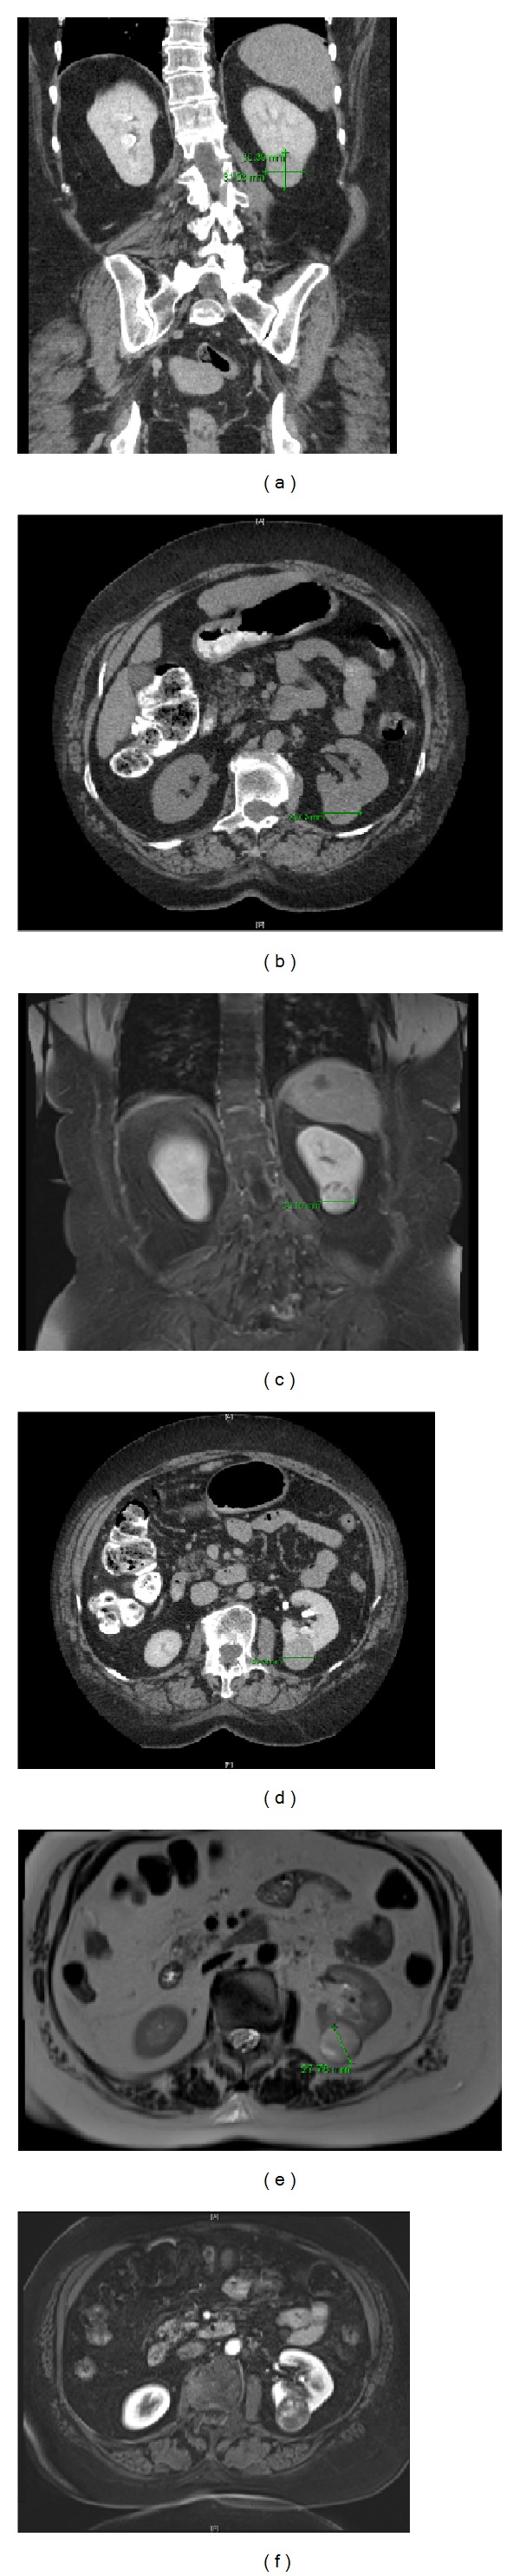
(a)–(c) CT revealed a solid tumor arising from the posterior cortex of the kidney near the inferior pole. The tumor measured 3.5 cm in greatest diameter. (d)–(f) MRI revealed an exophytic mass arising from the posterior cortex of the kidney near the inferior pole. The mass demonstrated pouch and its internal enhancement, heterogeneous high T2 signal, and restricted diffusion.
